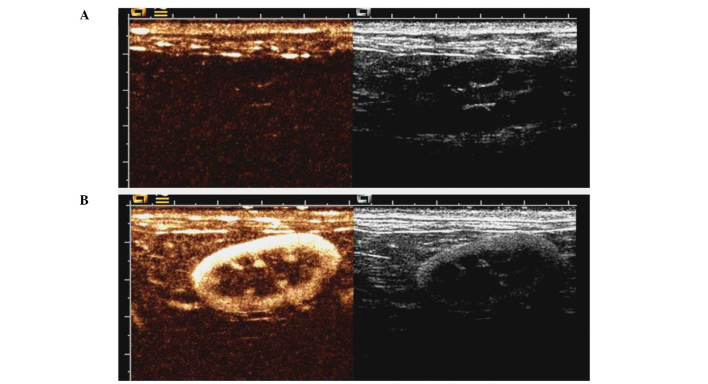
Representative *in vivo* ultrasound contrast-enhanced images in the rabbit right kidney. (A) Pre- and (B) post-administration of paclitaxel-poly(lactic-co-glycolic acid)-monomethoxy poly(ethylene glycol) nanocapsule solution. Left, contrast mode; right, conventional B-mode.
